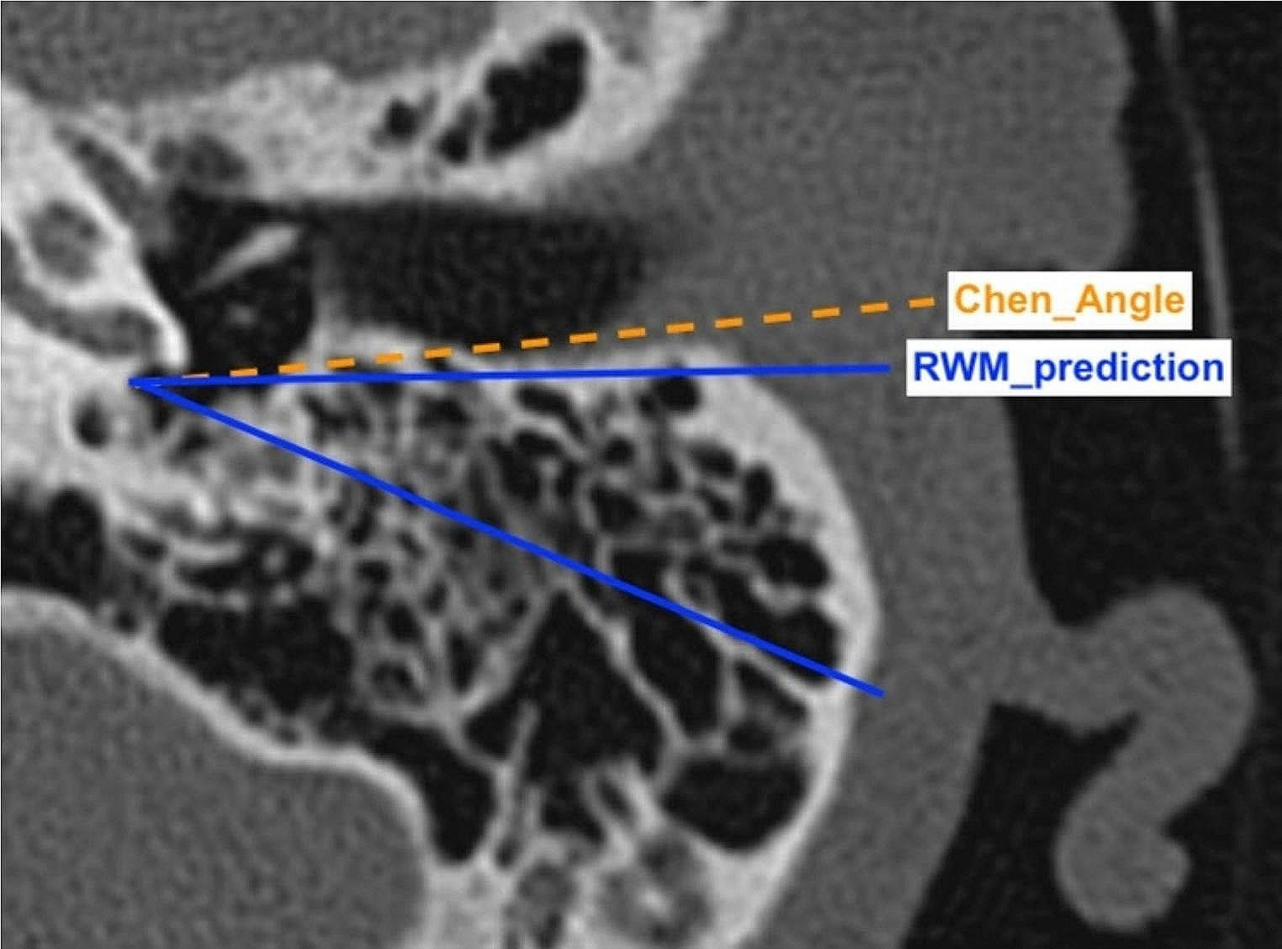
Computed tomography of the mastoid, left ear, axial plane. A comparison of the round window access measurements proposed by Chen et al. [22] - angular parameter A: the angle between the lower (solid blue) line and the (dashed orange) line labelled “Chen_Angle” and the measurement proposed by authors of the present study (RWM_prediction): the angle between the lower (solid blue) line and the (solid blue) line labelled “RWM_prediction”
